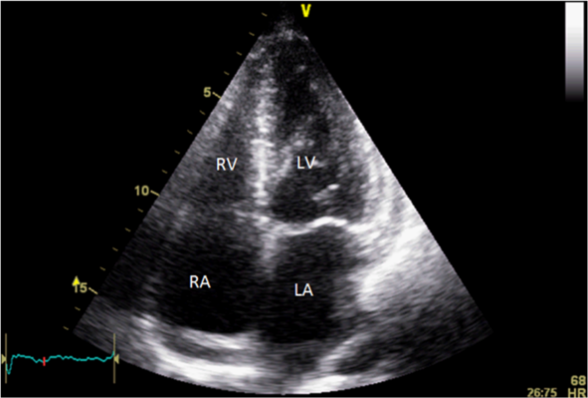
Is an apical four chamber view 2 weeks post pericardiocentesis and development of LV dysfunction showing resolution of the apical ballooning in systole with normal LV systolic function
